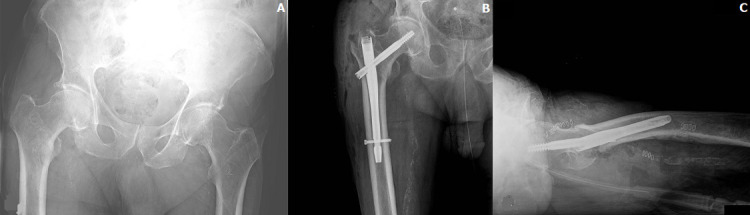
pre- (A) and post-operative (B, C) X-rays of a patient COVID positive with a fracture of the proximal femur
